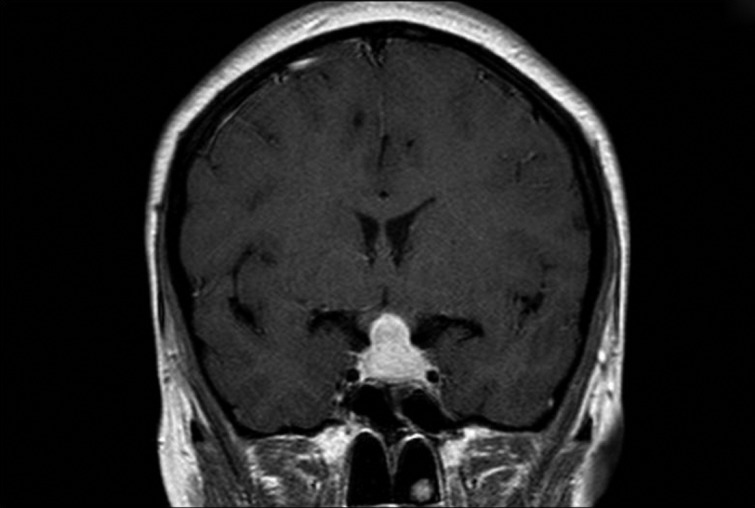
MRI scan of pituitary fossa at presentation.
